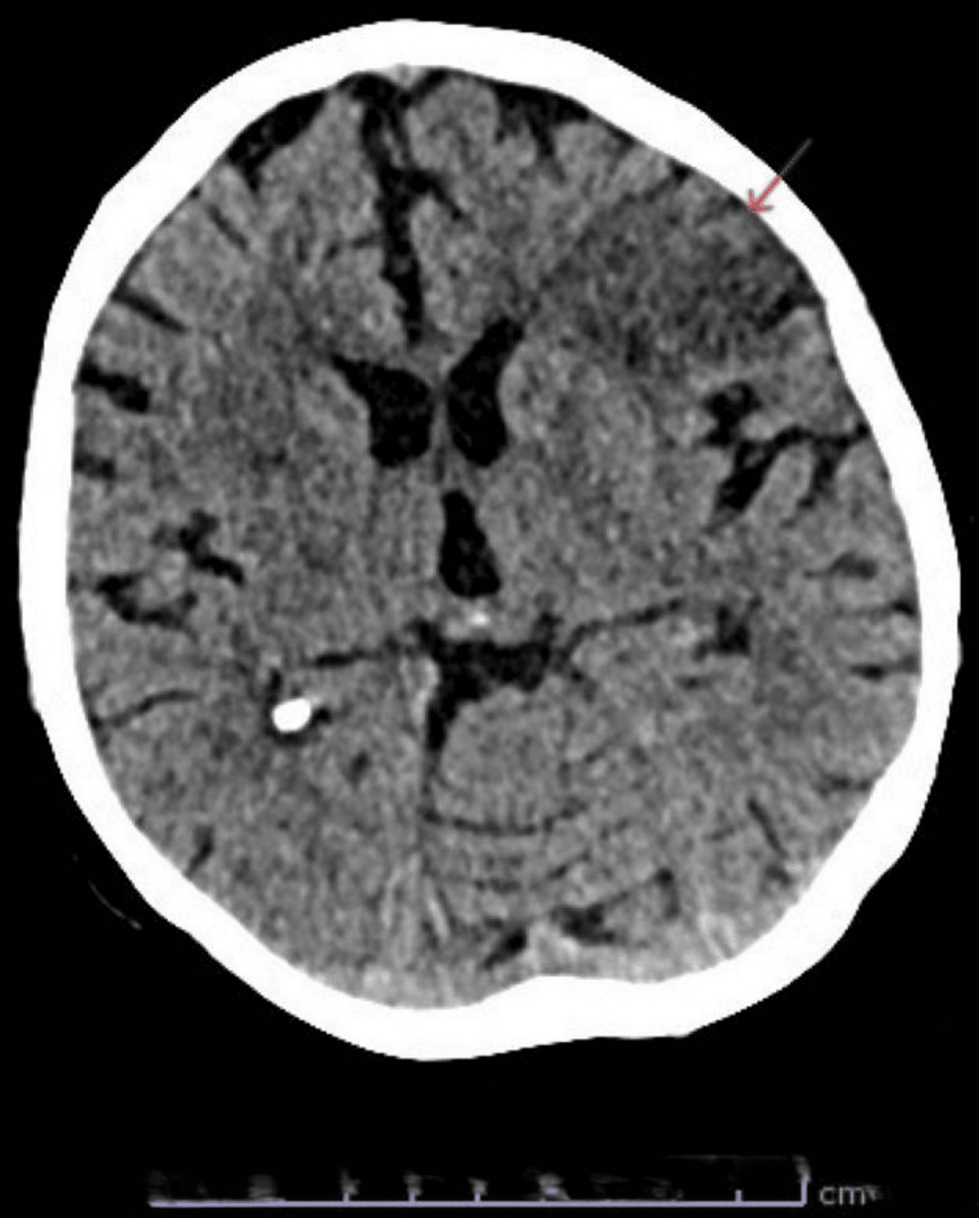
Initial non-contrast computed tomography of the head demonstrating a subacute infarct in the left frontal lobe, seen as hypodensity with a mild loss of gray-white matter differentiation (area of interest marked by arrow), corresponding to the patient’s presenting expressive dysphasia and right-sided weakness.
